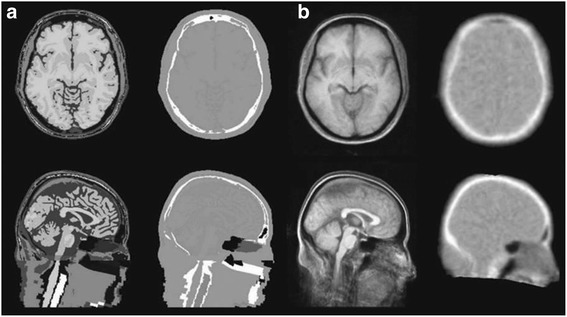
Transverse and sagittal sections through MRI and attenuation maps generated from the tissue atlas (a) and measured templates (b) (This research was originally published in *I. B. Malone, R. E. Ansorge, G. B. Williams, P. J. Nestor, T. A. Carpenter, and T. D. Fryer*, “Attenuation correction methods suitable for brain imaging with a PET/MRI scanner: a comparison of tissue atlas and template attenuation map approaches., (Malone et al. 2011)” J. Nucl. Med., vol. 52, no. 7, pp. 1142–9, 2011. © by the Society of Nuclear Medicine and Molecular Imaging, Inc.)
